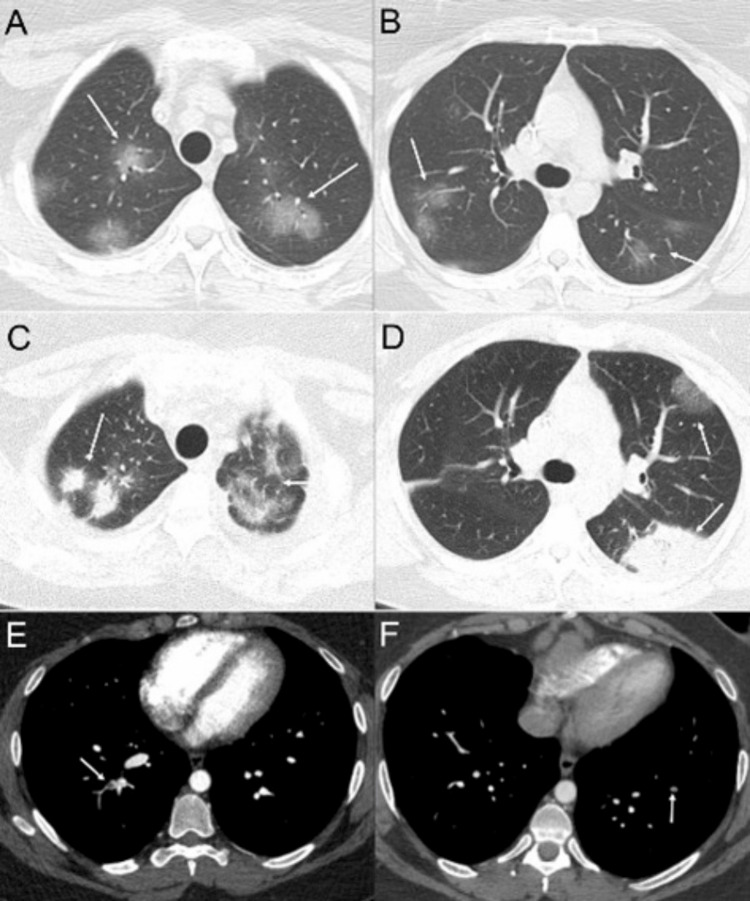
Eosinophilic granulomatosis with polyangiitis, diagnosed by a history of asthma, peripheral eosinophilia, paranasal sinus disease, and cutaneous vasculitis. Axial CT images (A and B) demonstrate bilateral, multifocal ground-glass opacities (arrows). These opacities are resolved on a subsequent follow-up CT. Axial images from a follow-up CT performed several years after the initial scan (C and D) reveal new multifocal ground-glass opacities and consolidations (arrows). Additionally, axial CT images from two different pulmonary CT angiography exams (E and F) show filling defects in the pulmonary arteries consistent with pulmonary emboli (arrows).
